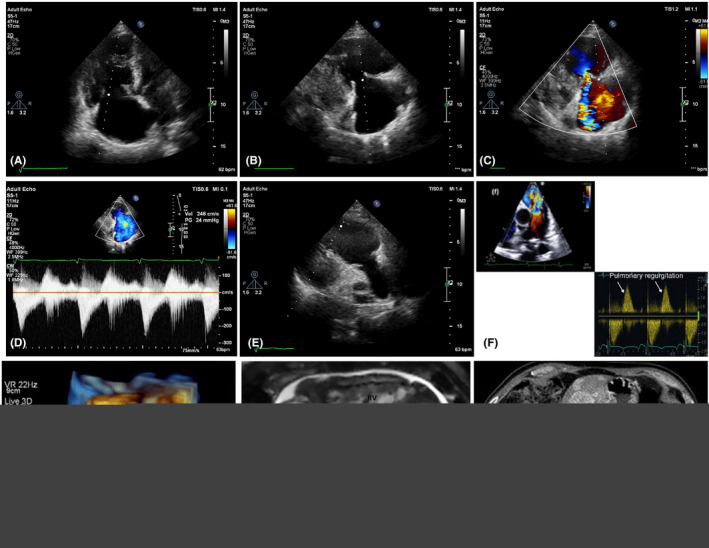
Carcinoid heart disease. A, TTE in the 4‐chamber view shows RV and RA enlargement with thickened, retracted anterior and septal TV leaflets, leading to the malcoaptation of the TV leaflets. B, RV‐inflow tract view in TTE shows thickened and shortened anterior and posterior leaflets on TV. C, Color Doppler study shows severe free tricuspid regurgitation. D, Doppler profiles shows classical “dagger‐shaped” and low‐pressure TR. E, Parasternal short‐axis view shows thickened, retracted TV leaflets. F, Doppler profile shows severe PI in color Doppler study with short PHT of PI. G, Three‐dimensional TEE shows thickened and malcoapted TV leaflets with a fixed systolic defect in systole. H, Cardiac MRI shows carcinoid involvement of TV leaflets. I, Multiple liver metastases of gastrointestinal tumors are visualized herein. TTE, Transthoracic echocardiography; RV, Right ventricle; RA, Right atrium; TV, Tricuspid valve; TR, Tricuspid regurgitation; PV, Pulmonary valve, PI, Pulmonary insufficiency; PHT, Pressure half‐time; MRI, Magnetic resonance imaging
